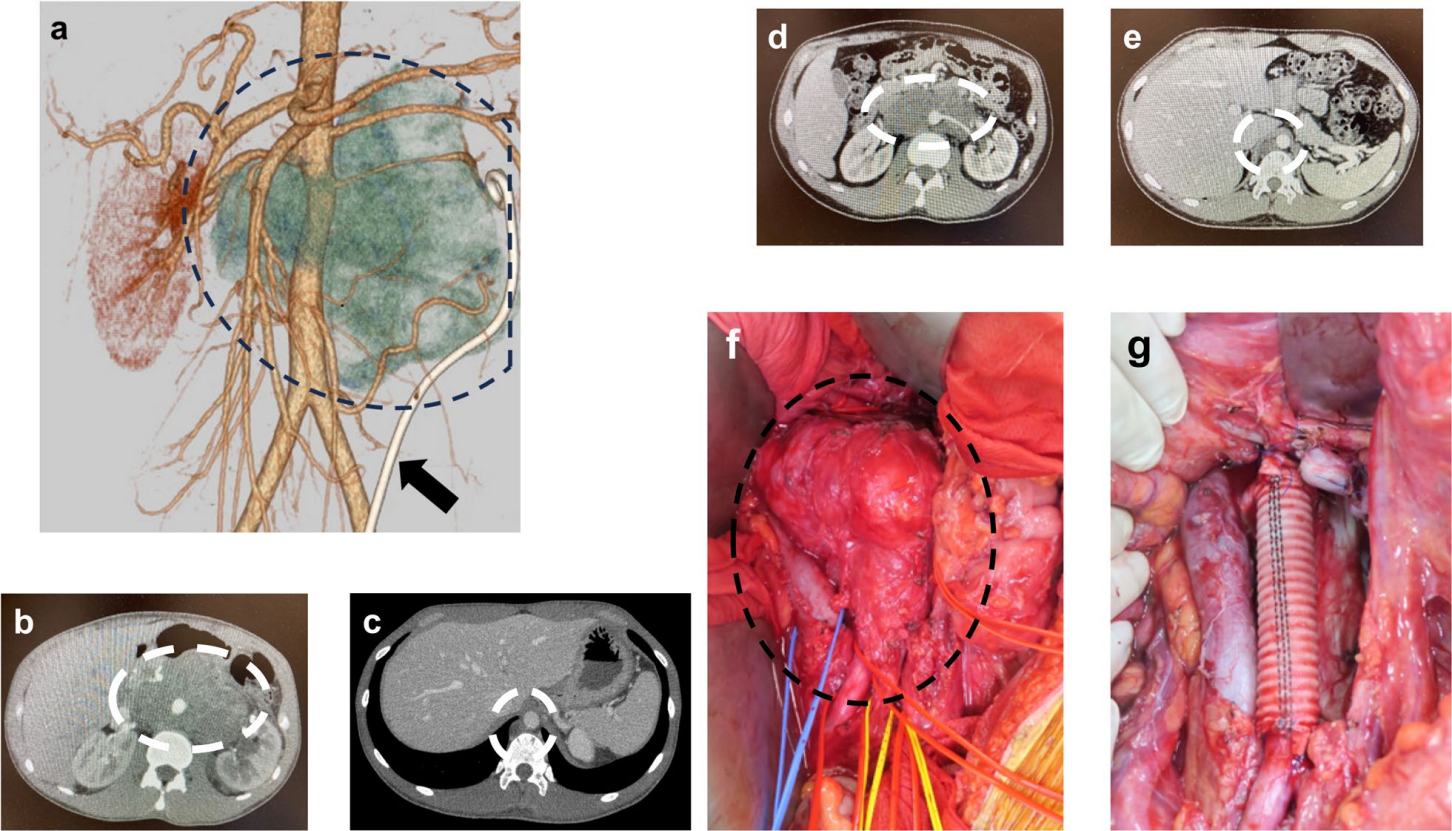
Computed tomography (CT) images and intraoperative findings. a The three-dimensional CT image captured before chemotherapy. The dotted line delineates the extent of the tumor. The black arrow indicates the ureteral stent. b, c CT images before chemotherapy. d, e CT images after chemotherapy. f Intraoperative findings. The dotted line indicates the extent of the tumor. g Intraoperative findings after tumor resection with abdominal aorta and reconstruction by prosthetic graft
